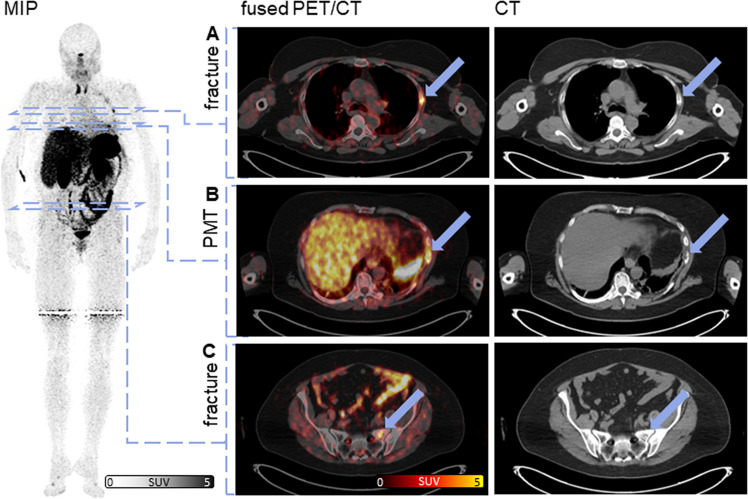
Example of a 63 year old patient (number 11) with osseous PMT (B: SUVmax 9.61) and bone fractures (A: SUVmax 4.32 and C: SUVmax 4.56), depicted in MIP, fused PET/CT and CT. *CT* computer tomography, *MIP* maximum intensity projection, *PET* positron emission tomography, *PMT* phosphaturic mesenchymal tumors, *SUV* standardized uptake value
